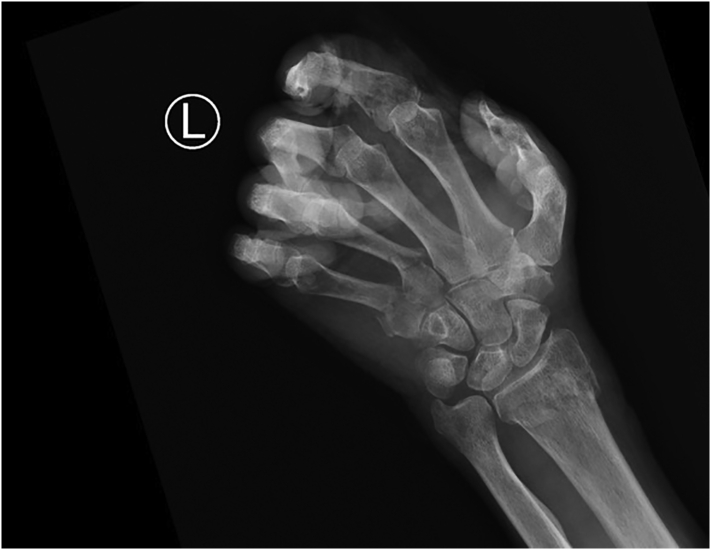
Posterior anterior radiograph of left hand showing numerous fractures.
